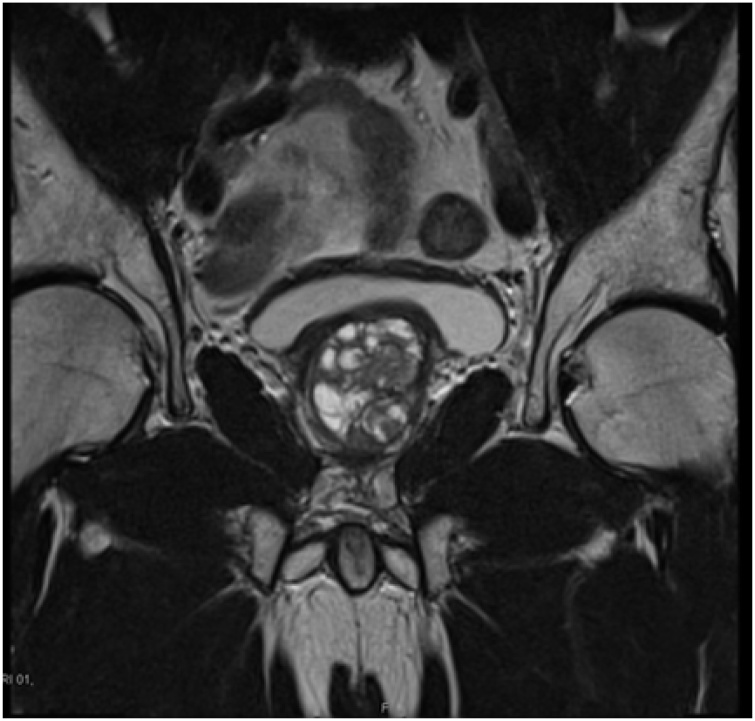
T2-weighted MRI scan (coronal view) shows enlarged prostate growing into the bladder base pre-TURP.
